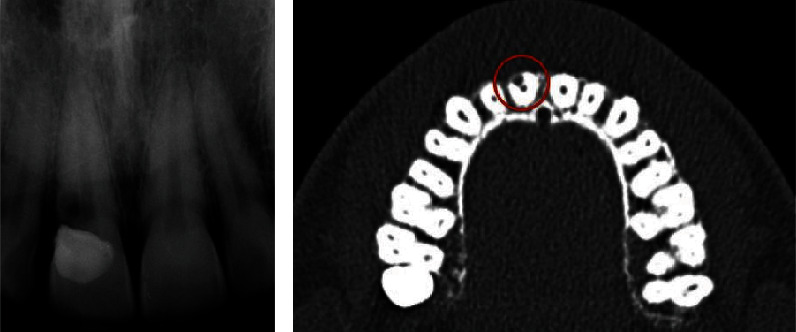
(a) Preoperative periapical X-ray and (b) preoperative CBCT.
